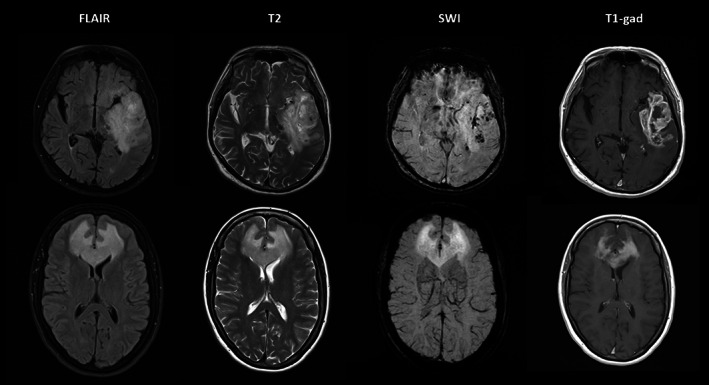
Top row: 74‐year‐old man presenting with aphasia was found to have a high‐grade left temporal glioma on MRI (1.5 T). Note the intratumoral susceptibility signals (ITSS) on SWI indicative of microhemorrhage and vessel proliferation. Bottom row: 57‐year‐old woman presenting with behavioral changes due to a lymphoma. SWI does not show any ITSS despite marked homogeneous enhancement.^62^
